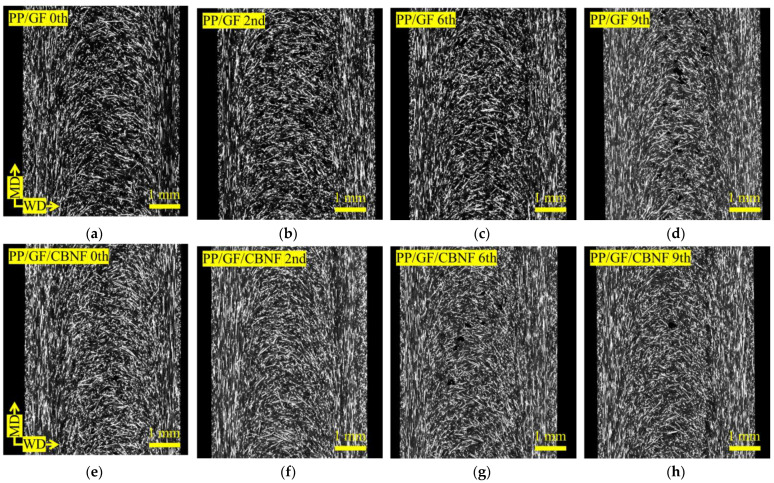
X-ray CT images of the core region. Images (a–d) correspond to PP/GF, and images (e–h) to PP/GF/CBNF, after 0, 2, 6, and 9 extrusion cycles.
